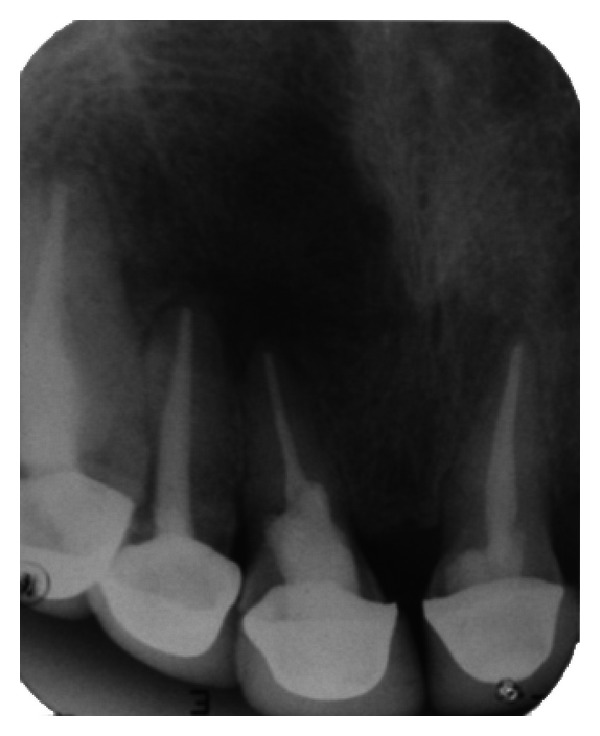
24-month followup IOPA radiograph.
